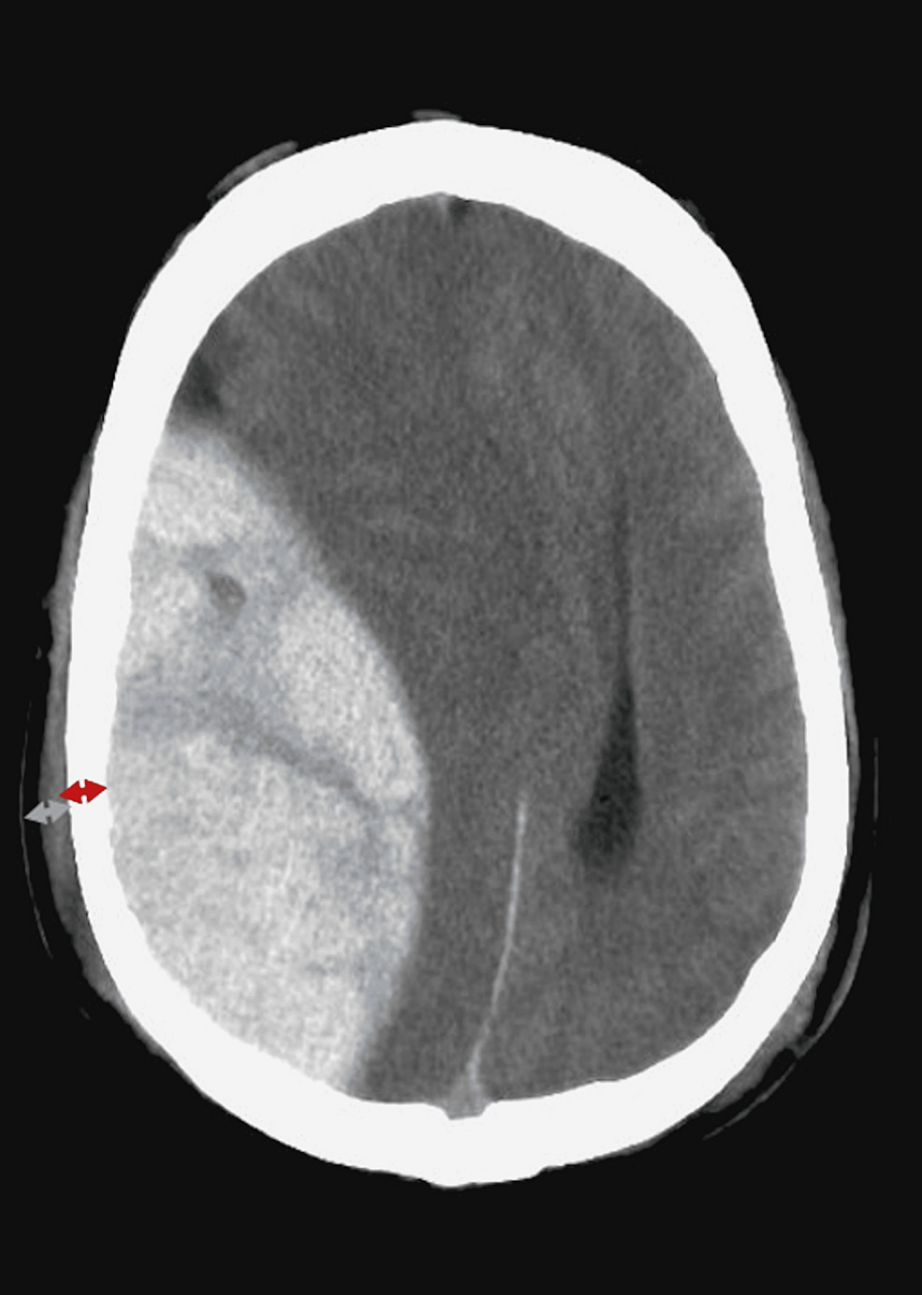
Non-contrast brain CT depicting epidural hematoma. The skull thickness (red arrow) should be measured in order to select the appropriately sized intraosseous needle to ensure skull penetration and to avoid injuring the parenchyma. If the scalp incision is not made, the scalp thickness (gray arrow) should also be taken into account when selecting the intraosseous needle. Case courtesy of Associate Professor Frank Gaillard, www.radiopaedia.org.
